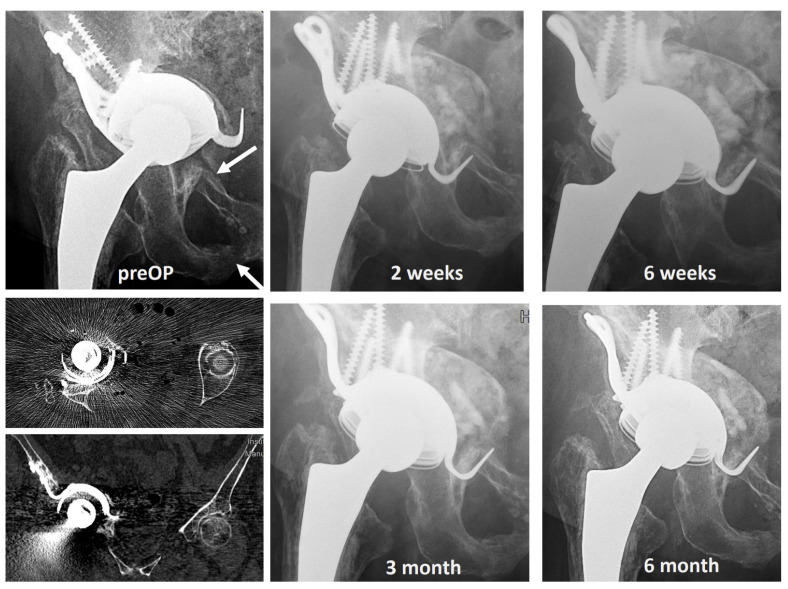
Radiographic 6-month follow-up of a 76-year-old female with a critical size pelvic defect (pelvic discontinuity) after failed revision cup and fracture of the os ischium and superior pubic ramus. The arrows show the fracture gap. The large pelvic defect was treated by bioactivated 2 × 20 cl TCP granules (5000–8000 µm) as well as an allograft, and the hip joint was reconstructed by a cementless, modular revision titanium cup (MRP, Fa. Brehm, Germany). Three months postoperative the patient achieved full weight-bearing. The latest follow-up showed a healed fracture and an osseous integrated cup.
